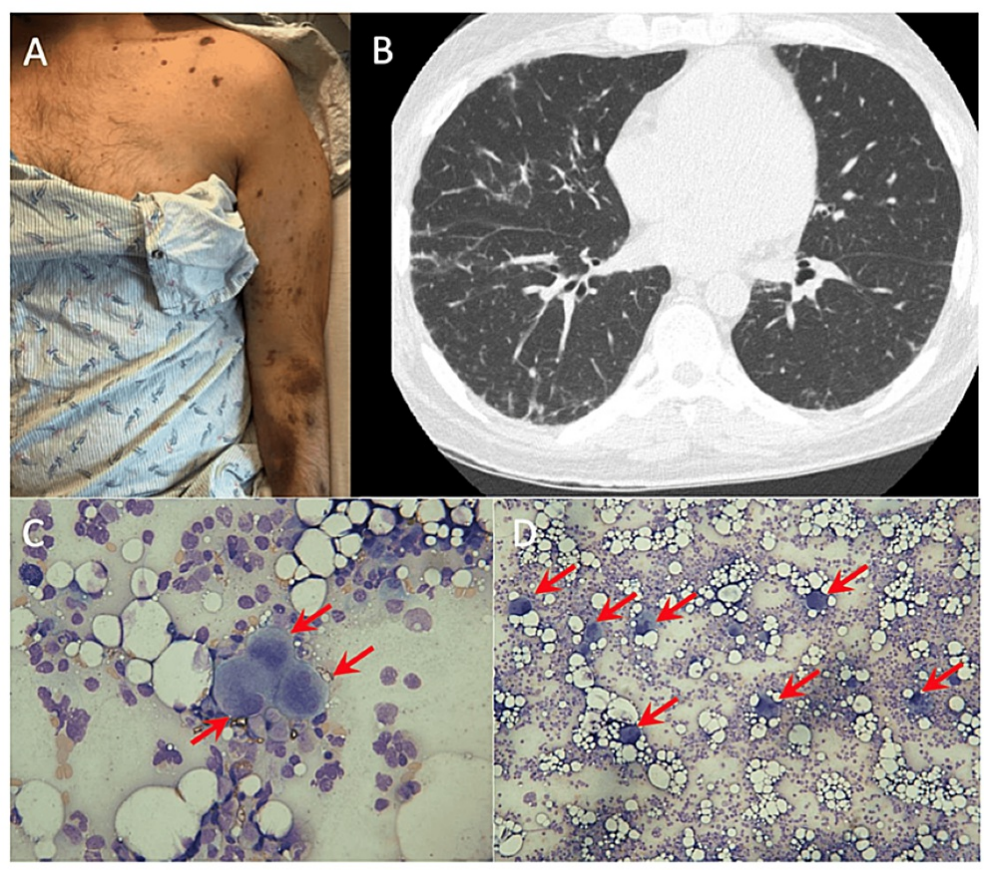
Patient skin exam, imaging, and peripheral blood smear (A) Photograph of the patient’s left arm on initial presentation demonstrating multiple ecchymoses. (B) High-resolution lung CT scan demonstrating fibrotic non-specific interstitial pneumonia that can be seen in the setting of myositis-related ILD. (C) and (D) Bone marrow aspirate smear (600× and 430× magnification, respectively) demonstrating adequate megakaryocytes (arrows). CT, computed tomography; ILD, interstitial lung disease.
